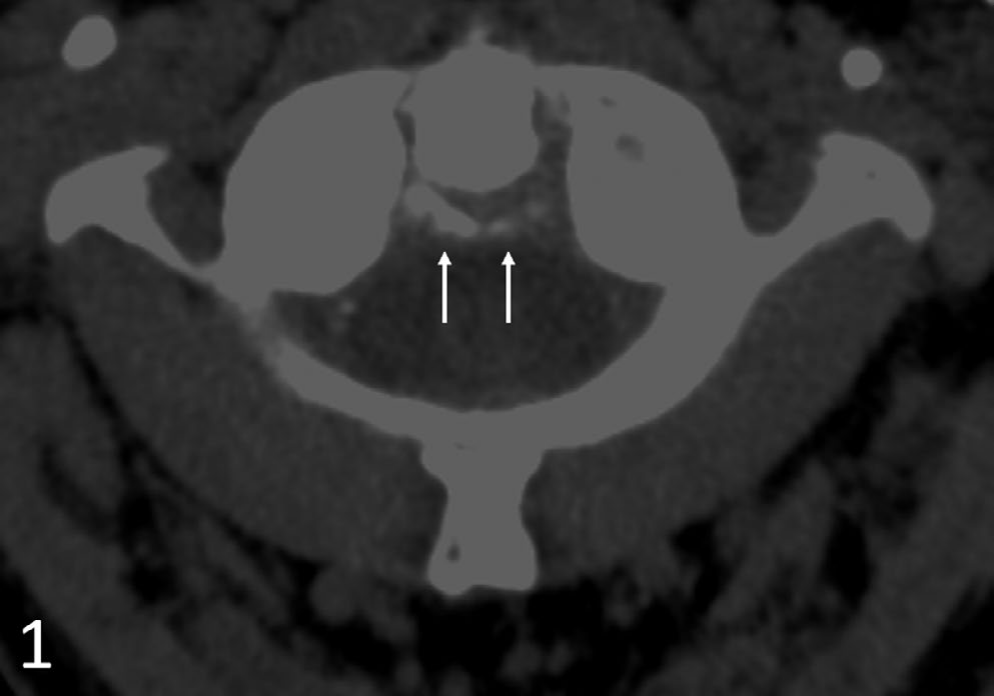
Axial CT of the cervical spine indicating linear calcifications of the transverse ligament of the atlas
